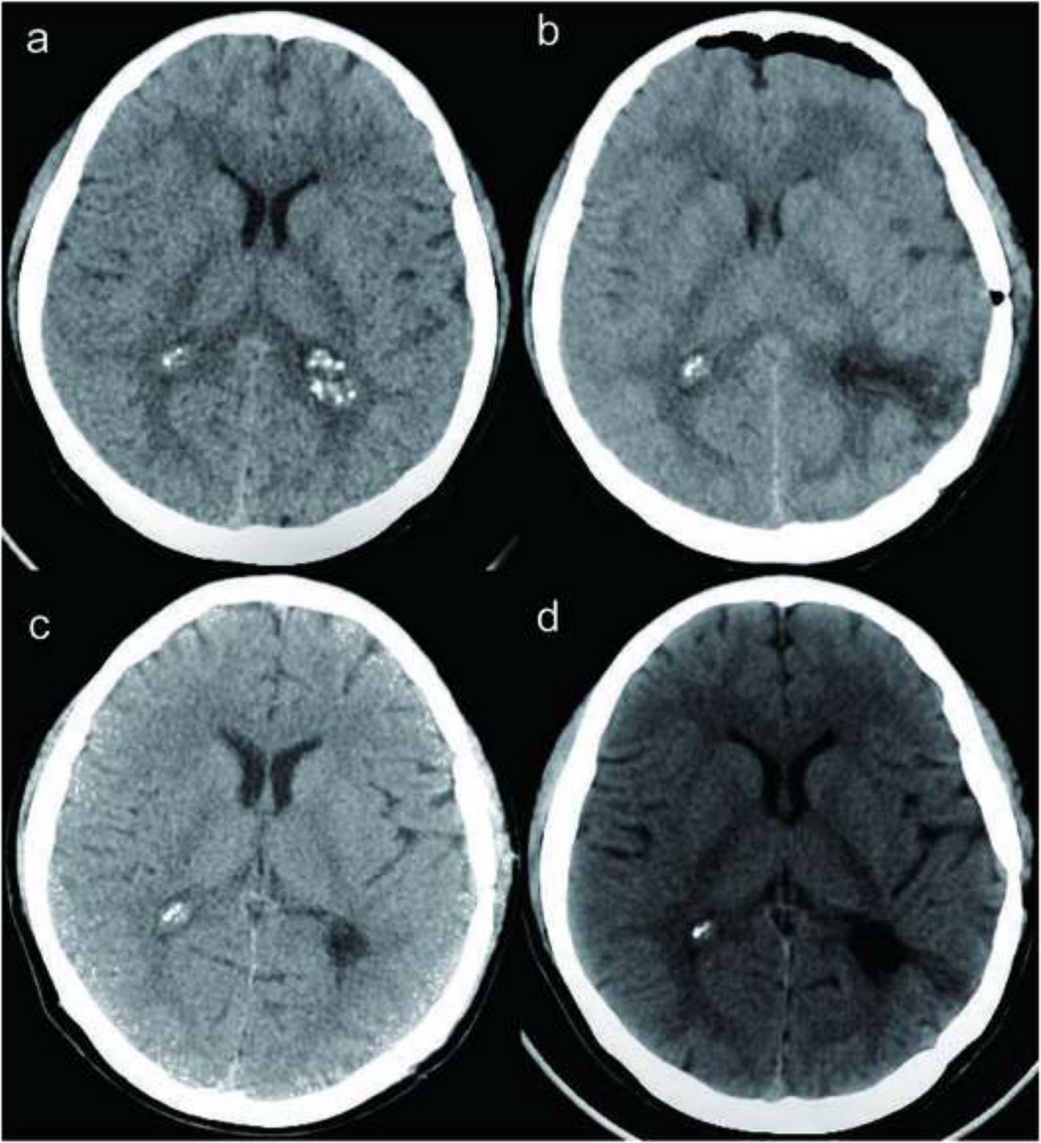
Presents CT scans of the head before and after surgery. The high-density lesion on the left side was successfully removed without any residual calcification. a The preoperative head CT scan. b–d Head CT scans taken at 3 days, 1 month, and 27 months after surgery, respectively. It is evident that the left mass was completely eradicated with no signs of recurrence, while the right mass remained unchanged in size
